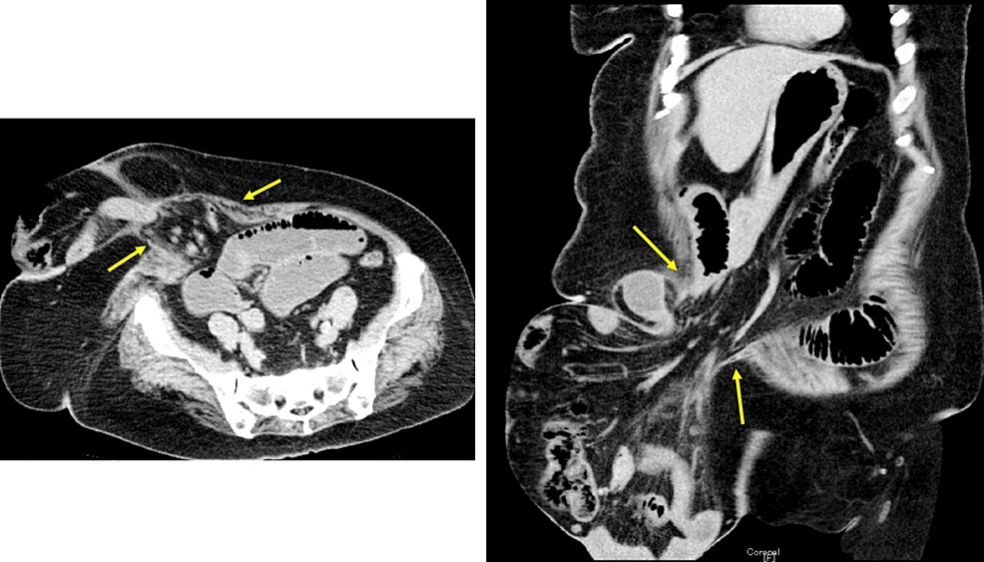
CT scan findings in axial plane (left) and coronal plane (right) CT scan showed herniation of the terminal ileum and ascending colon in the umbilical hernia, with small intestine dilation oral to the hernia. The hernia orifice measured 8 cm (arrow). CT: computed tomography
